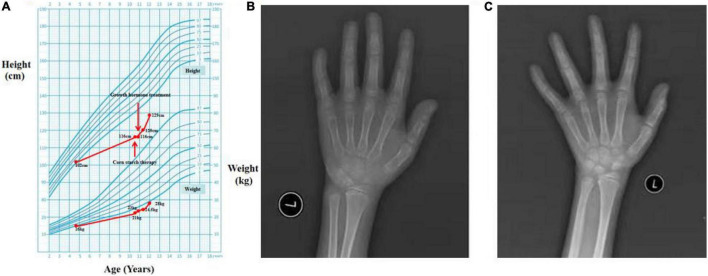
The growth curve and bone ages. (A) The growth curve of Chinese boys (8). (B,C) Plain X-rays of the left hand and wrist.
